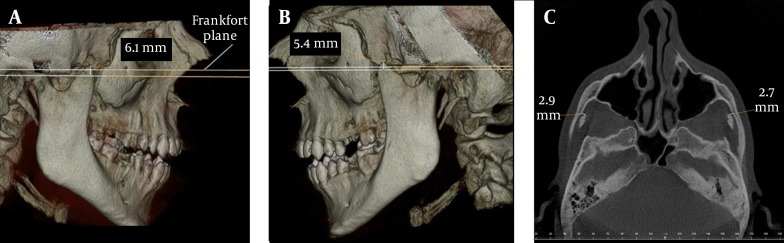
Case 1; A, 3D view of the elongated right coronoid process; B, 3D view of the elongated left coronoid process; C, The distance between the coronoid process and the inner face of the frontal part of the zygomatic bone on axial CBCT scan
